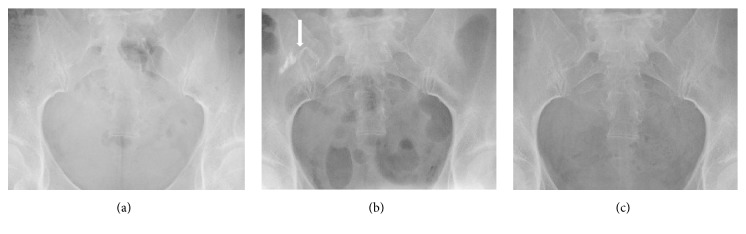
Abdominal X-ray test. (a) Preoperative X-ray test. Findings were within normal limits. (b) Postoperative X-ray test. A cord-like material was captured in the lower right abdomen. (c) X-ray test one month after operation. The cord-like material disappeared.
